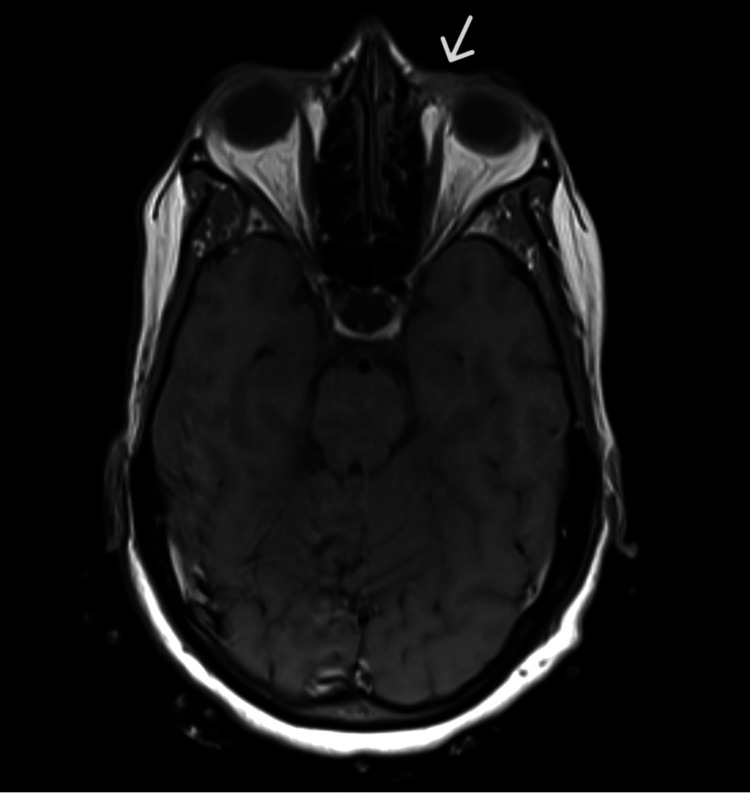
An MRI image showing a lesion in the left lower eyelid (without IV contrast)
